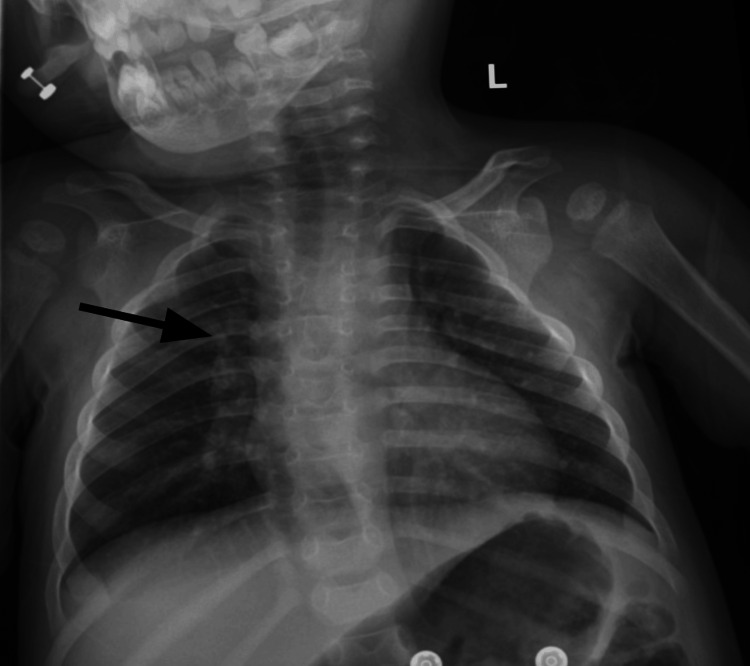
The patient’s chest X-ray on her third day of hospitalization demonstrated perihilar and peribronchial thickening, again consistent with bronchiolitis (black arrow).
